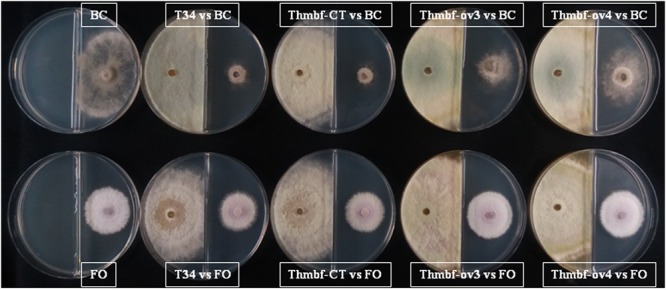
Dual cultures of strains T34, Thmbf-CT, Thmbf-ov2, Thmbf-ov3 and Thmbf-ov4 of *T. harzianum* and the pathogens *B. cinerea* (BC) and *F. oxyxporum* (FO) on discontinuous PDA medium. Plates only with the pathogen were used as controls. All plates were incubated at 28°C for 5 days.
